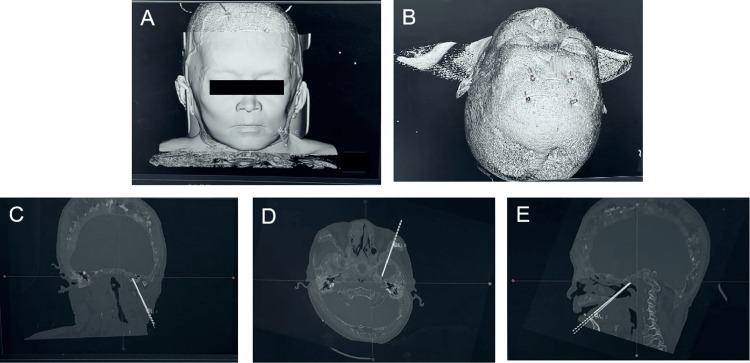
Intraoperative clinical images Registration was performed using four bony landmarks with the robotic pointer. The surgical plan, based on fused MRI and CT images, determined the needle path angles in the coronal (C), horizontal (D), and sagittal planes (E).
